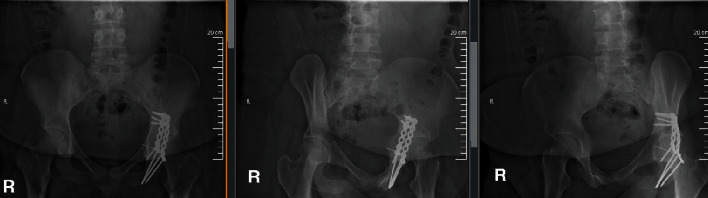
Postoperative AP and Judet radiographs of the pelvis demonstrating stable internal fixation and progression of healing at three months following surgery.
